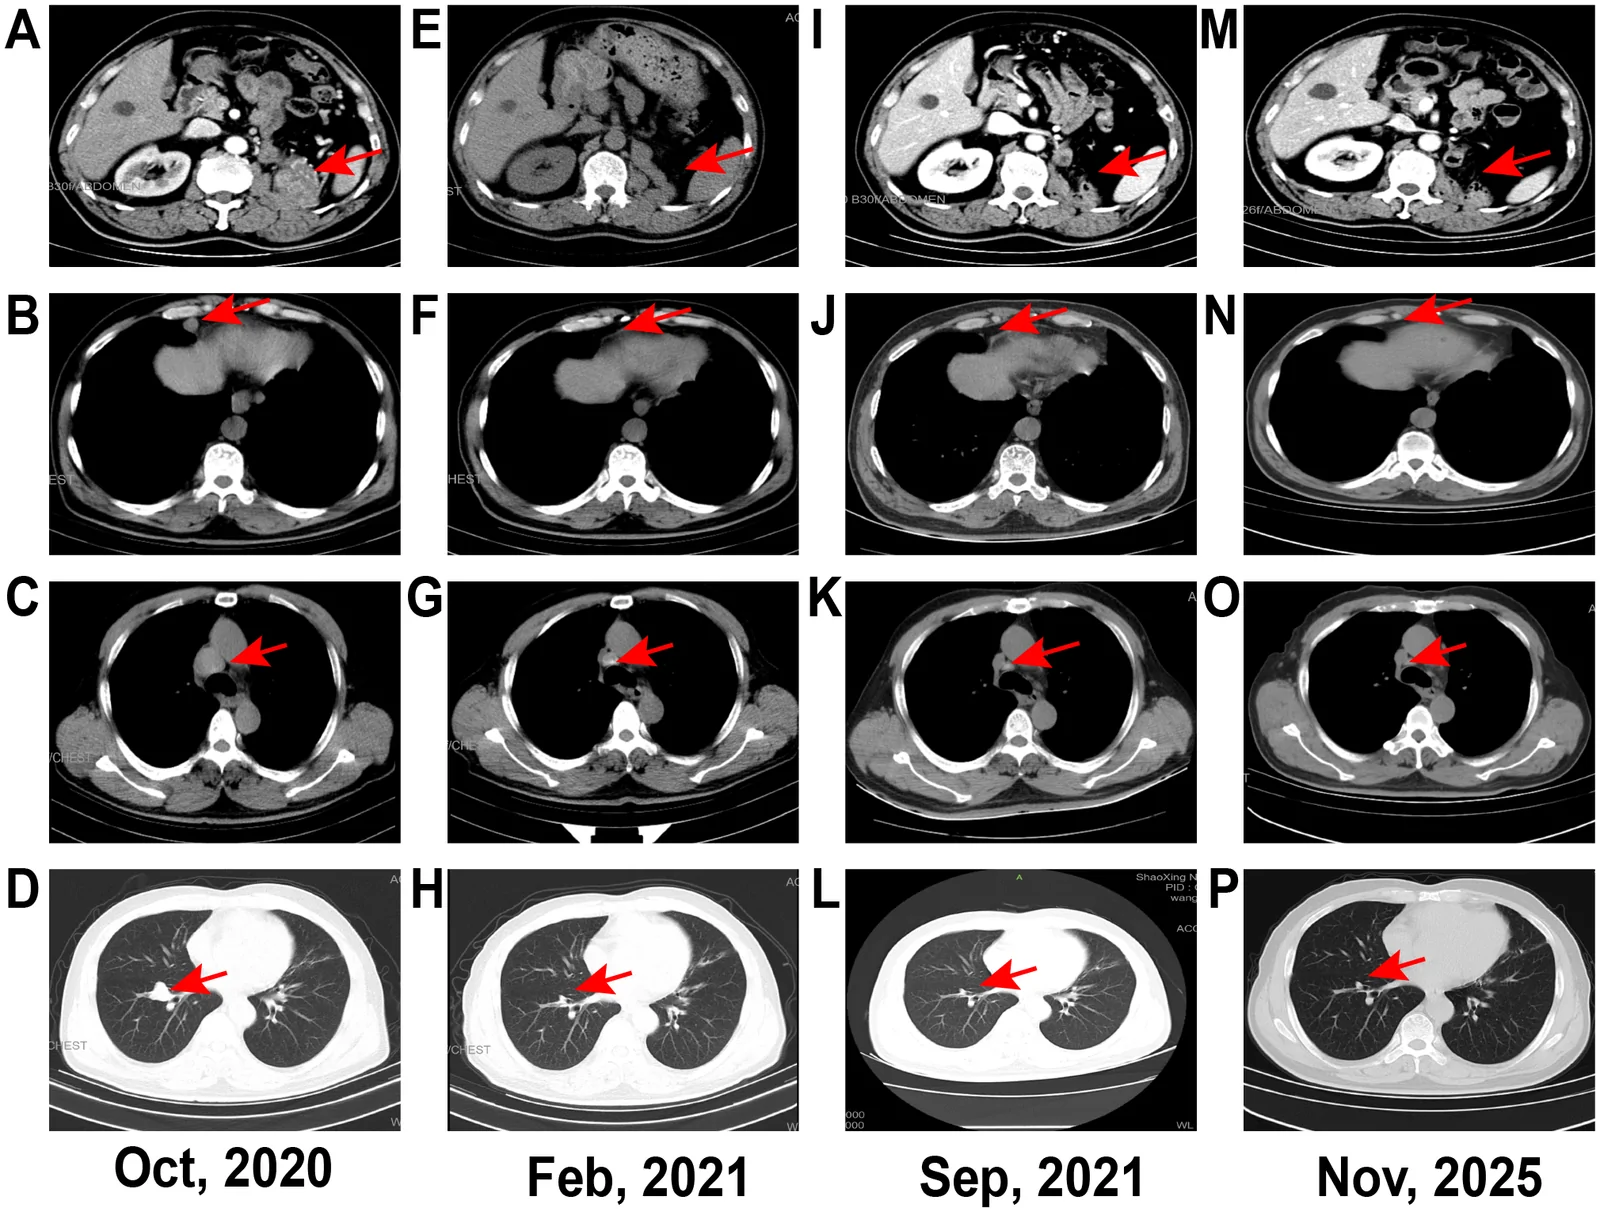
Tumor volume changes after third-line therapy assessed using CT. (A–D) CT scans performed in October 2020, prior to initiation of third-line therapy with toripalimab and axitinib. (A) An irregular, soft-tissue mass measuring approximately 65 mm × 40 mm was identified in the left renal region; enhancing nodules were also observed in the retroperitoneal regions, as indicated by the red arrow. (B) A nodule at the cardiophrenic angle. (C) Mediastinal lymphadenopathy. (D) Pulmonary nodules. (E–H) CT images acquired in February, 2021. (E) A substantial decrease in the volume of soft tissue mass in the left renal region. (F) A marked reduction of nodele in cardiophrenic angle. (G) Regression of mediastinal lymph nodes. (H) Reression of the size of pulmonary nodules located in the right upper lobe. (I–L) CT images performed in September, 2021. (I) A marked reduction in the size of the left renal lesion. (J) No abnormalities in cardiophrenic angle (J). (K) No abnormalities in mediastinal lymph nodes. (L) No abnormalities in the right lung. (M–P) CT performed in November 2025 demonstrated no radiological evidence of disease.
